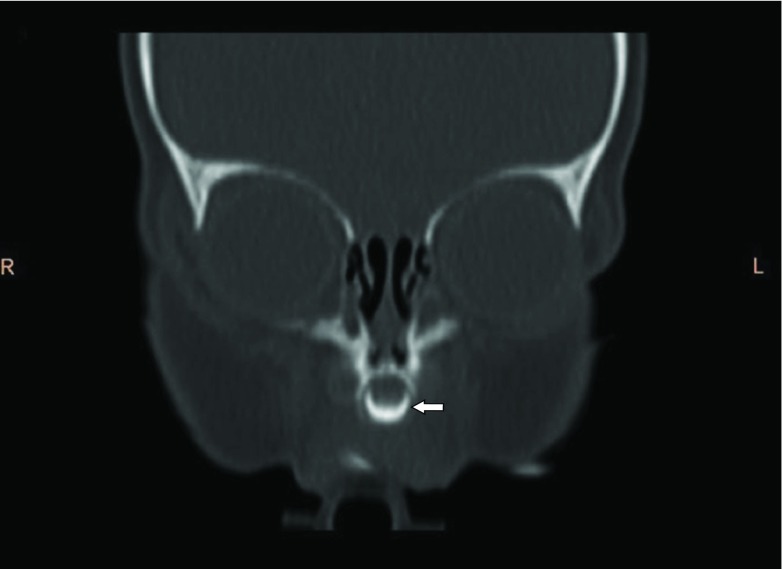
CT image (pre-stenting): coronal view showing central mega-incisor (white arrow).
